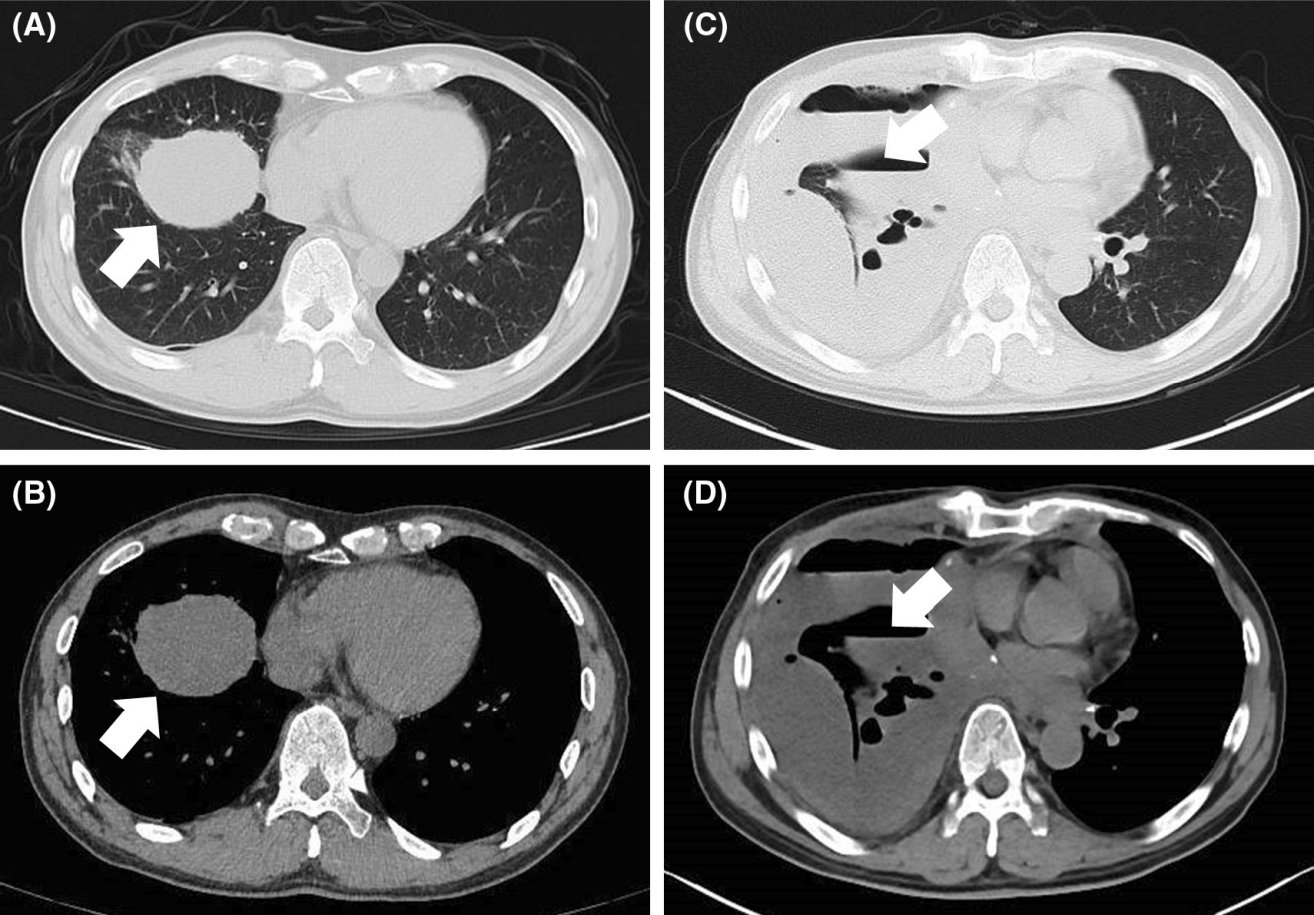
The features of chest CT image corresponding to the patient's condition. (A) A larger pulmonary nodule was obviously detected as arrow shown in the lung window. (B) A larger pulmonary nodule was detected as arrow shown in the mediastinal window. (C,D) The right pleural effusion was accompanied by the formation of air‐fluid level, which was partially encapsulated, and the right lung was infected with atelectasis after the first operation as arrow shown.
